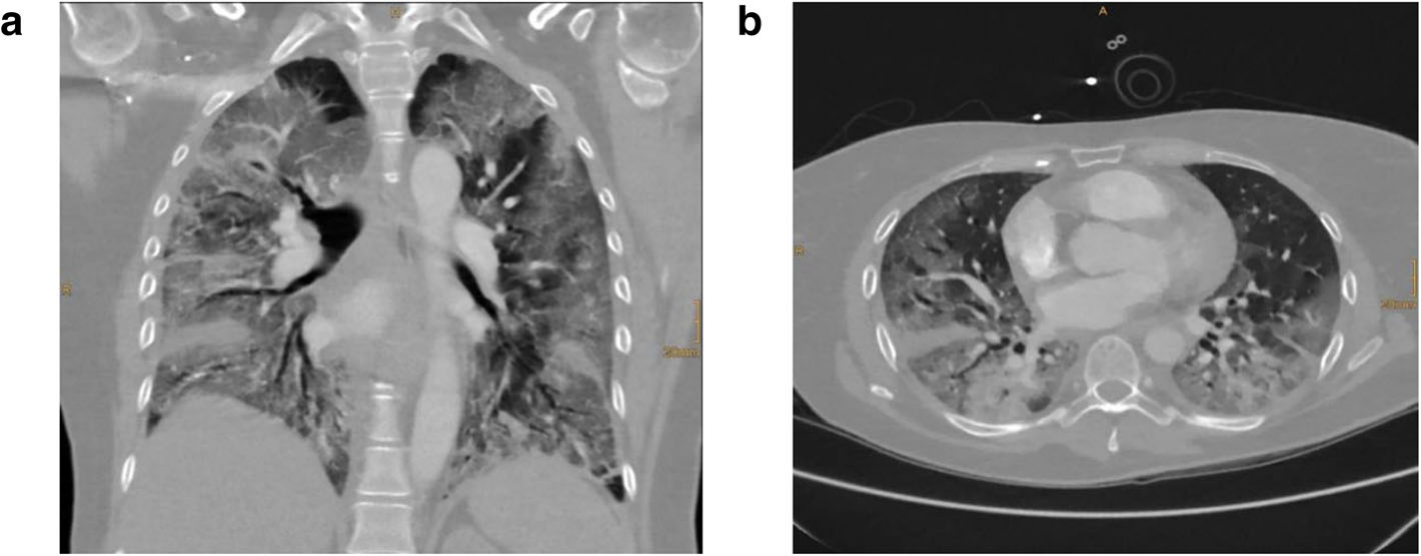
Computed tomography four days prior to admission showing spacious areas of ground glass opacities including consolidation and air bronchogram (a, coronal plane; b, axial plane, with kind permission of the Department of Radiodiagnostics, Central Hospital Bolzano, Bozen/Bolzano, Italy)
